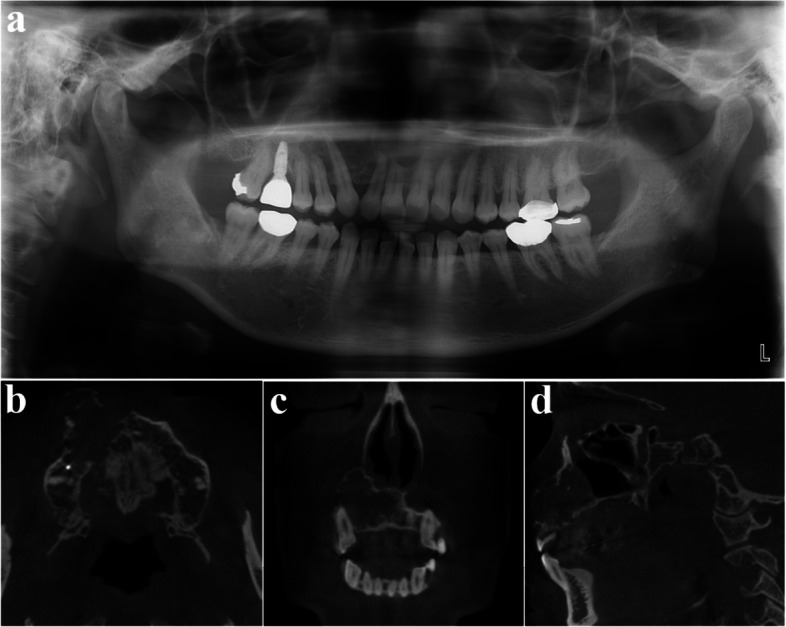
Preoperative radiographic images of a 53-year-old female patient with ameloblastoma in the right maxilla with displacement of multiple teeth, (a) panoramic radiograph, (b) CBCT, axial view, (c) CBCT, coronal view, (d) CBCT, sagittal view
